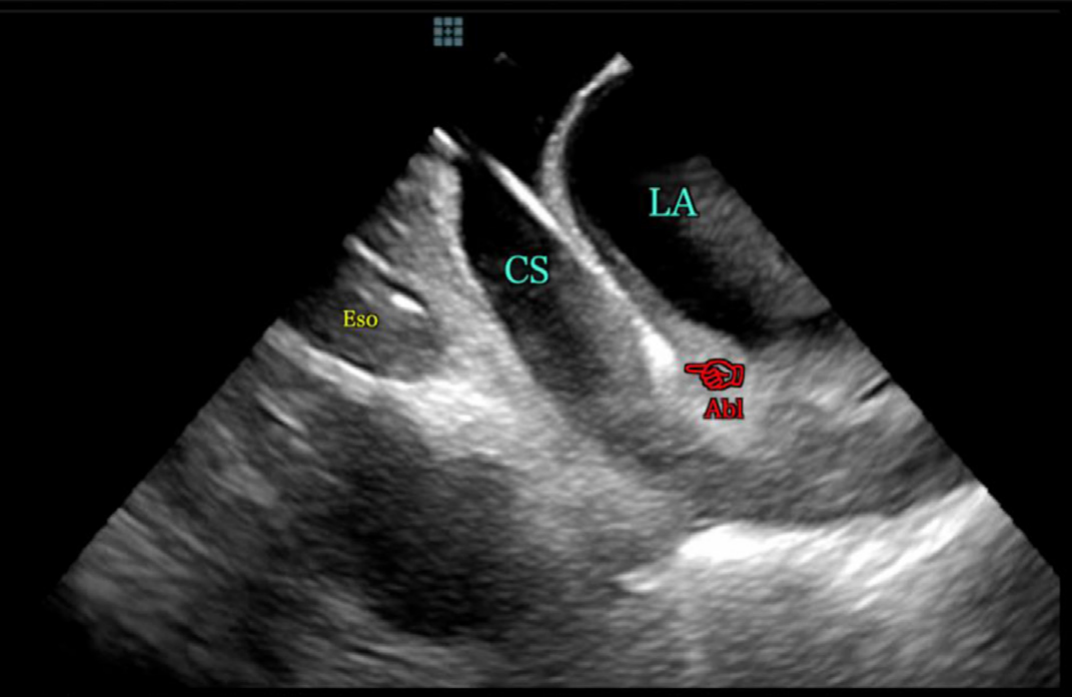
Intracardiac Echocardiographic Image Showing Ablation Catheter Within the Coronary Sinus Abl = ablation catheter; CS = coronary sinus; Eso = esophagus; LA = left atrium.
